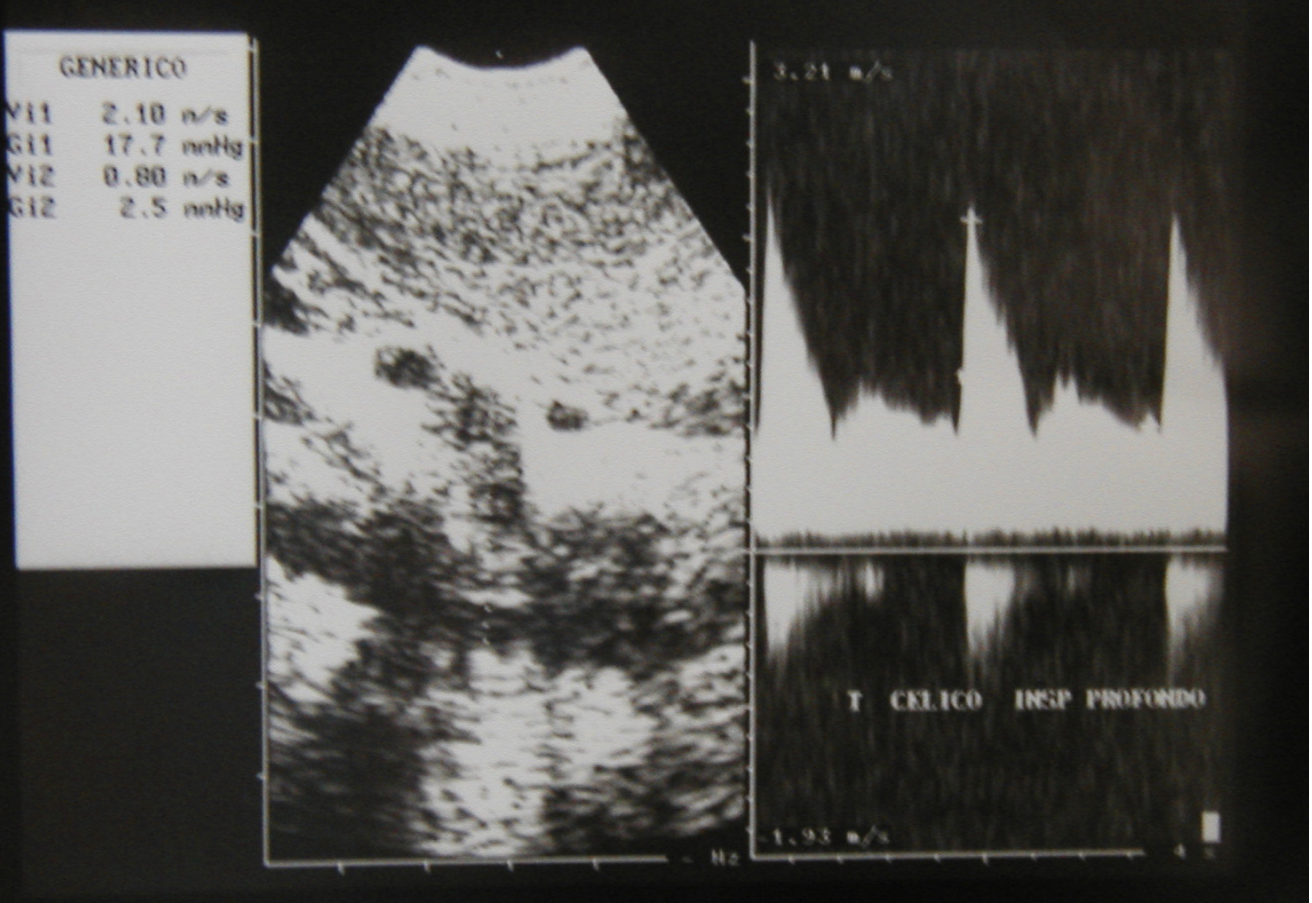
Continuous Doppler of Celiac Artery Stenosis, deep inspiration.
